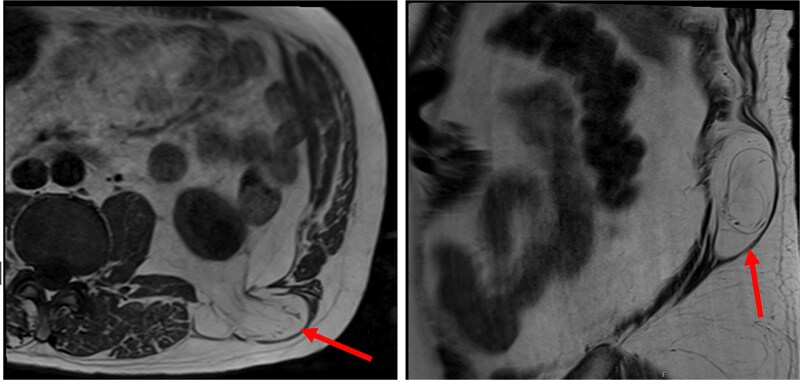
The patient’s MR imaging, which clearly shows the superior lumbar hernia. Axial section (left), sagittal section (right).
